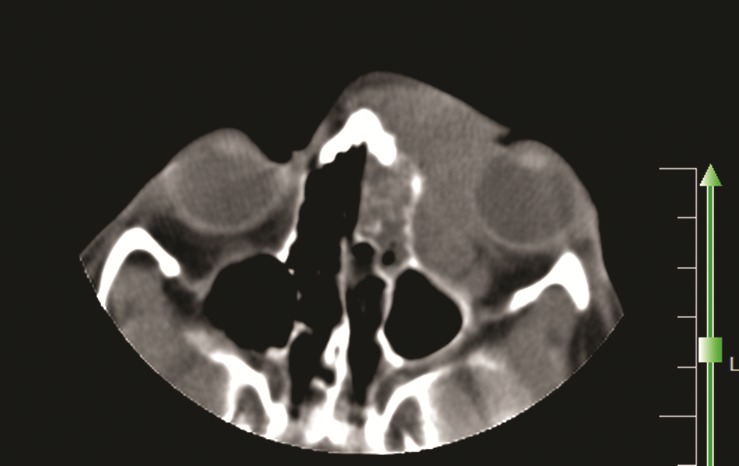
Figure 1: CT scan demonstrated opacity in the left maxillary, ethmoidal air cells, frontal sinuses and on the medial aspect of the extra-conal of the left orbit with moderate mass effect of the lesion on the left glob.
